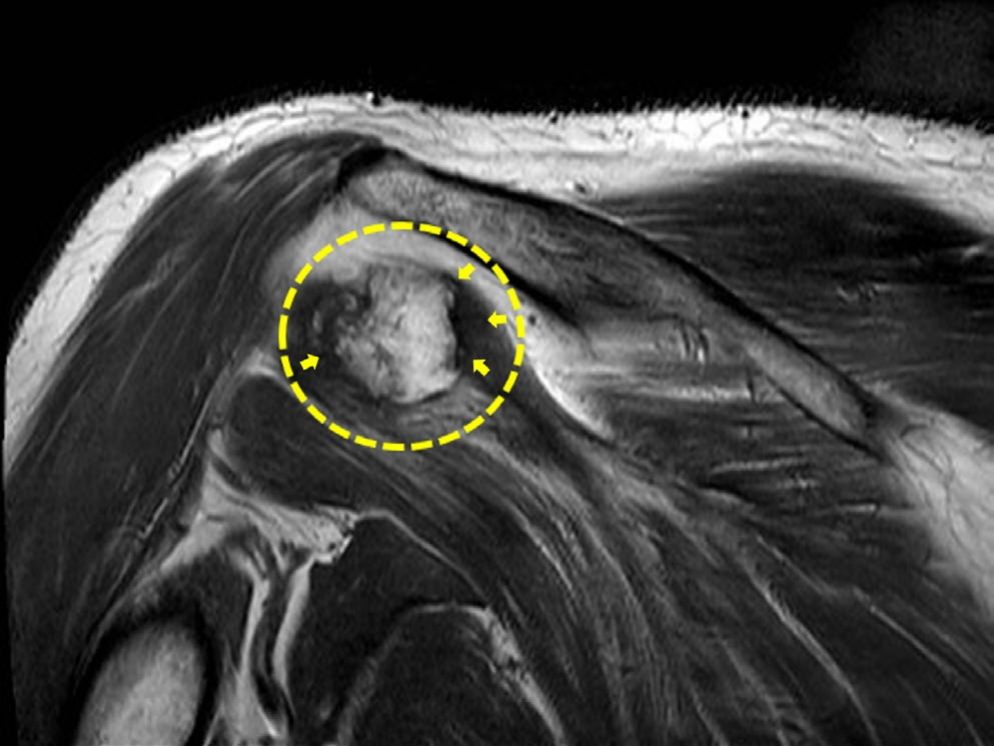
Coronal T1‐weighted MR image demonstrating a round inhomogeneous mass with signal intensity similar to fat, within in the infraspinatus muscle and consistent with a pattern of characteristic mature lamellar bone (yellow circle). A mild, low‐signal, peripheral rim is evident (yellow arrows). No surrounding edema is observed
